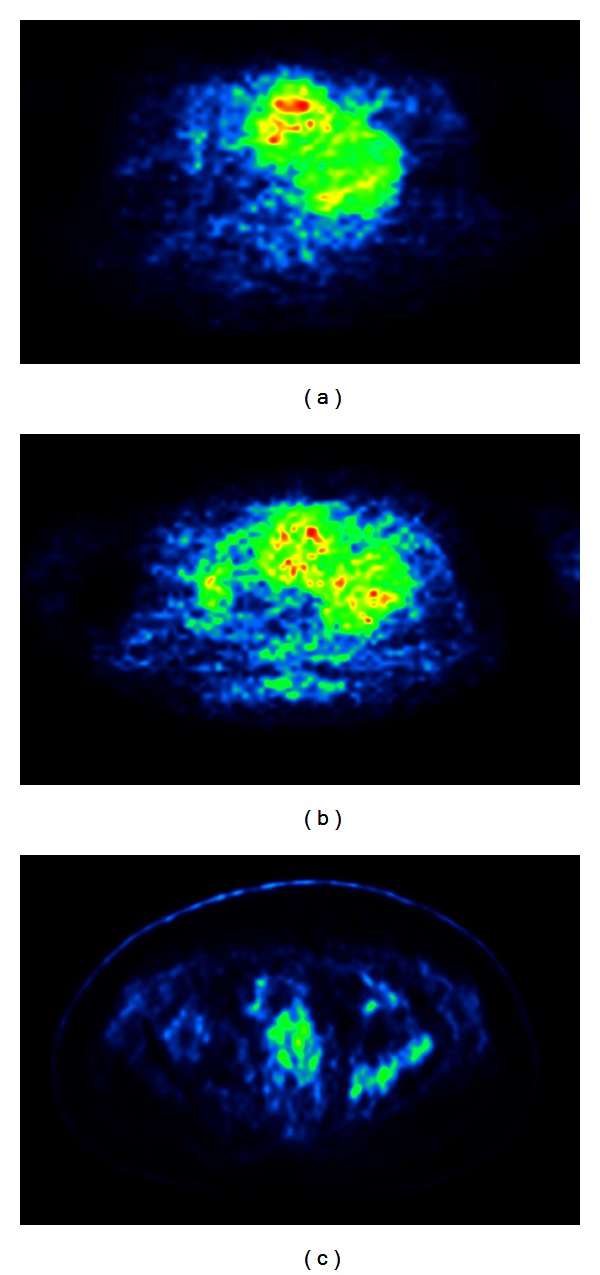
A 31-year-old female with a retroperitoneal desmoid tumor (case 3, Table 2; Figure 2) diagnosed in 2006 was treated with imatinib 800 mg daily. The FDG PET prior therapy with imatinib showed an average SUV of 4.2 and an SUV_max_ of 8.1 (a). After one month of imatinib treatment, the FDG PET demonstrated a decrease of the average SUV to 3.3 (−22%) and of the SUV_max_ to 6.1 (−25%) (b). Follow-up PET examinations in 2011 and 2012 (c) did not show any pathological FDG uptake. The corresponding conventional MRI documented PR according to RECIST.
